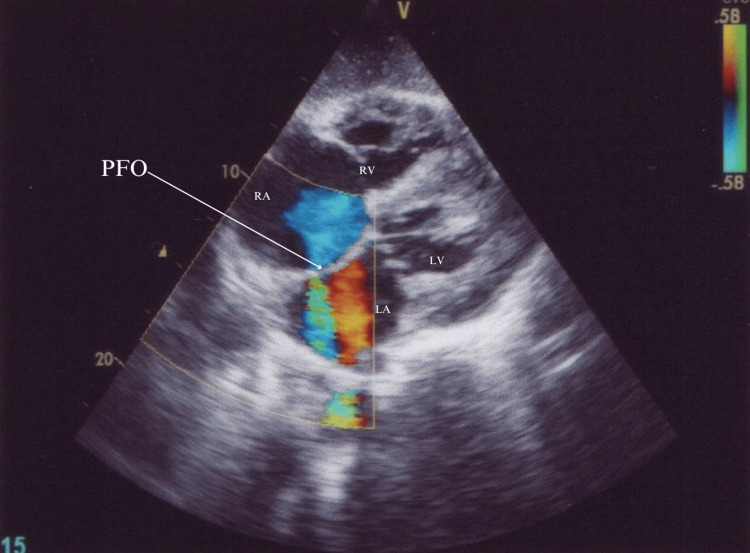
Echocardiogram suggesting the presence of a patent foramen ovale (PFO). RA: right atrium; RV: right ventricle; LA: left atrium; LV: left ventricle
